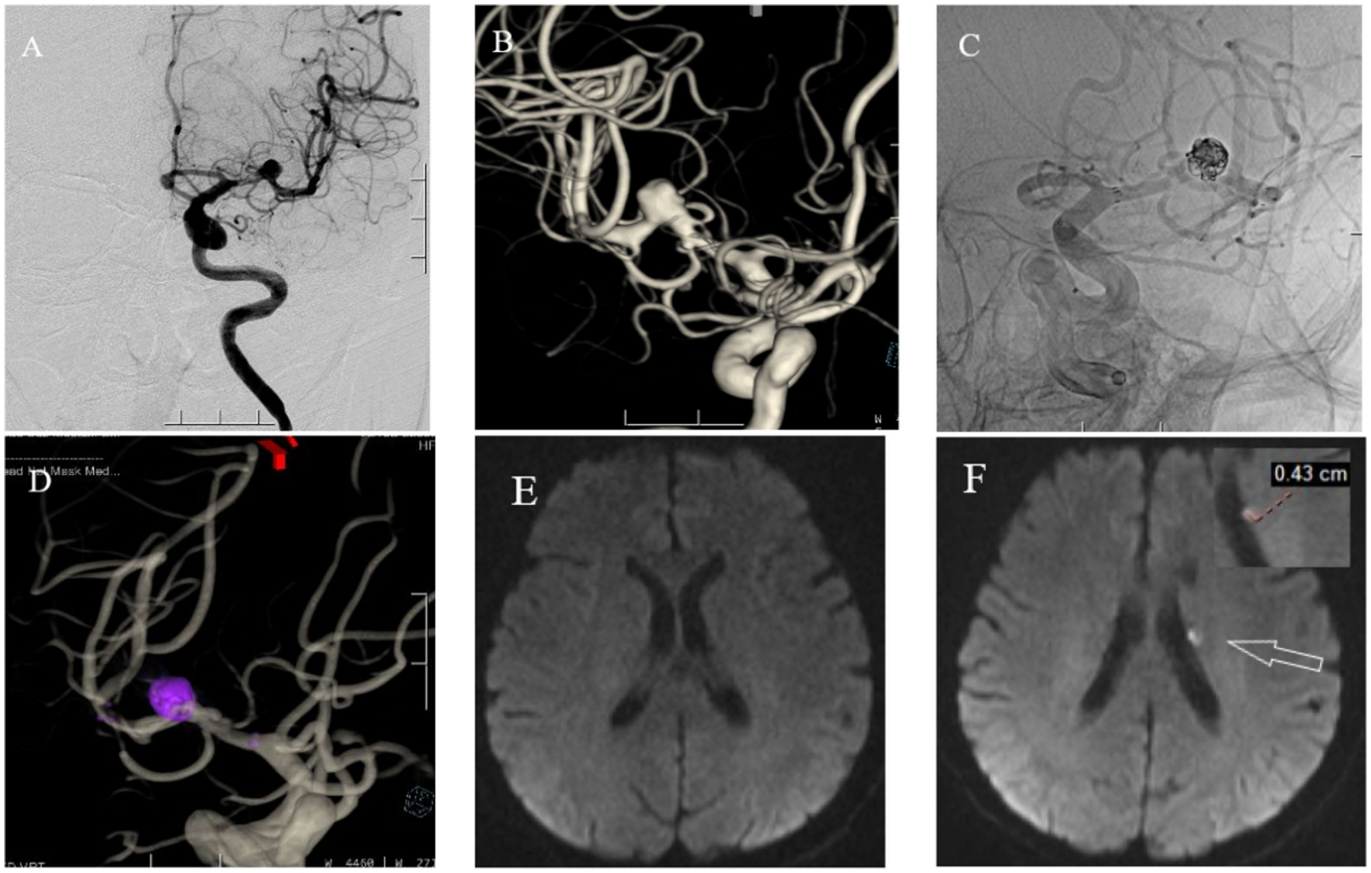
A 71 year old female with headaches, dizziness, and transient fainting for half a month. (A) Cranial DSA showing the location of the intracranial aneurysm in the 2D plane during the operation. (B) Cranial DSA showing a 3D image of the left middle cerebral artery aneurysm. (C) Intraoperative 2D plane showing the placement of coils and stents. (D) Postoperative 3D plane showing the placement of coils and stents. (E) Preoperative MRI-DWI showed no new infarcts. (F) Postoperative MRI-DWI on day three showed an acute microinfarction lesion in the left periventricular region with a diameter of approximately 0.43 cm.
